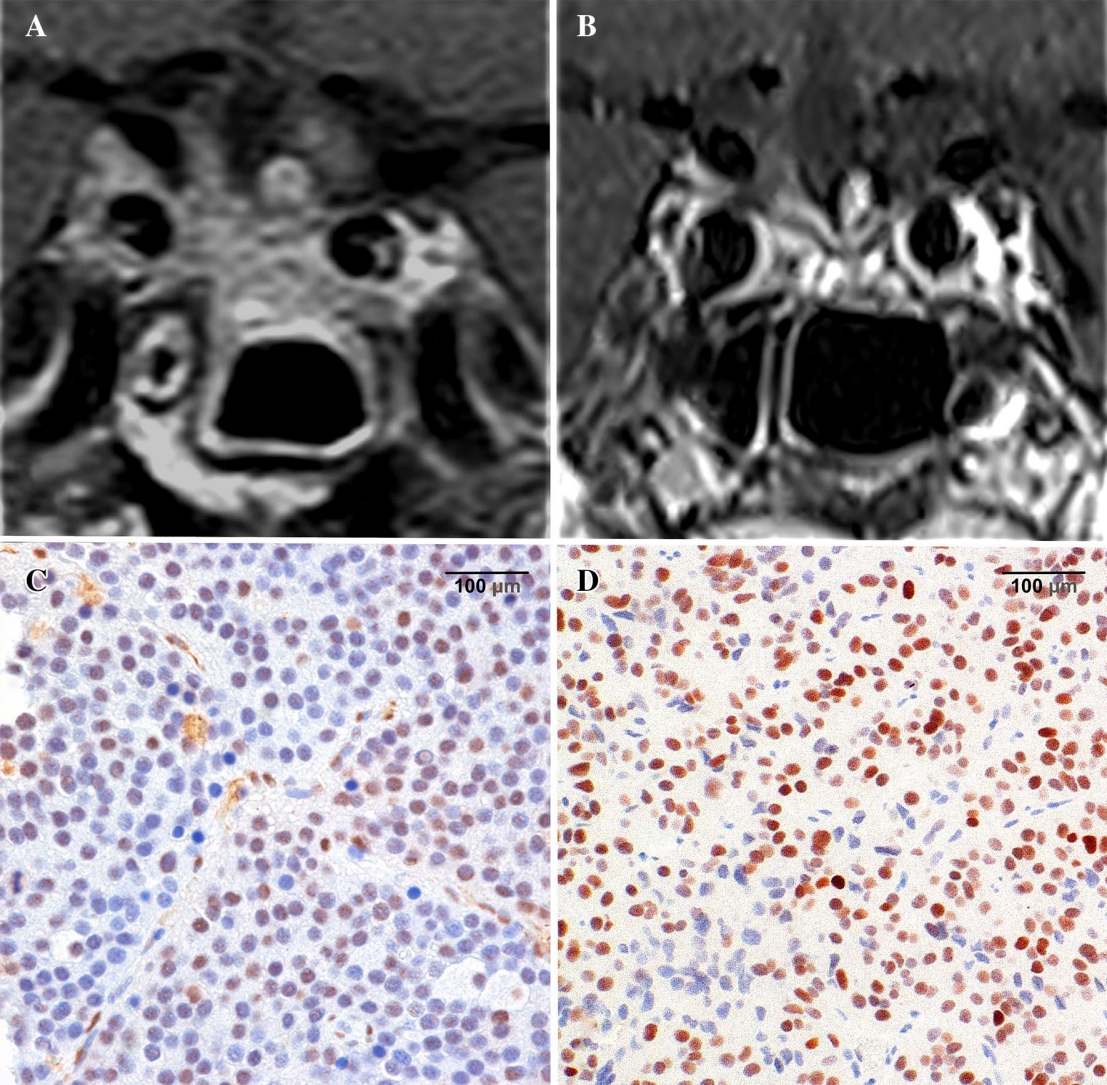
TMZ responder—invasive silent corticotroph adenoma subtype 1 in a 58-year-old male patient. a Coronal MRI at the start of TMZ treatment shows adenoma recurrence despite multimodal treatment (multiple transsphenoidal and subfrontal operations, pasireotide therapy). b Coronal MRI 6 months after the first TMZ cycle; although discontinued by the patient after 3 months due to side effects, a marked response to the TMZ treatment was found during follow-up and basal cortisol levels dropped to the normal range. c MGMT IHC sample from the last operation before TMZ treatment shows moderate MGMT immunoexpression (25–50%) ×40 magnification. d MSH6 IHC sample from the last operation before TMZ treatment shows high MSH6 immunoexpression (>50%) ×40 magnification
